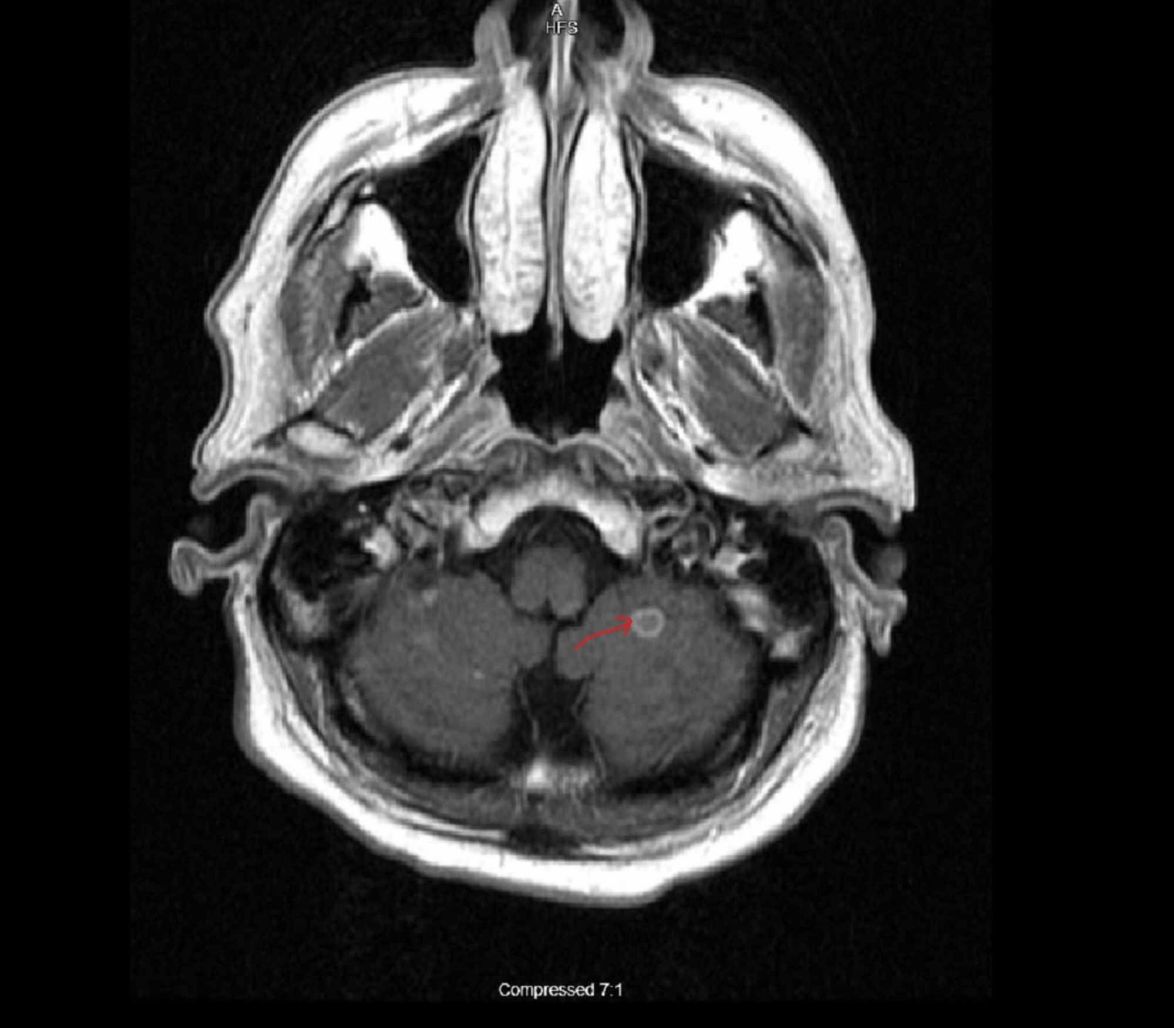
MRI brain showing ring enhancing lesion in cerebellar hemisphere (Arrow pointing)
